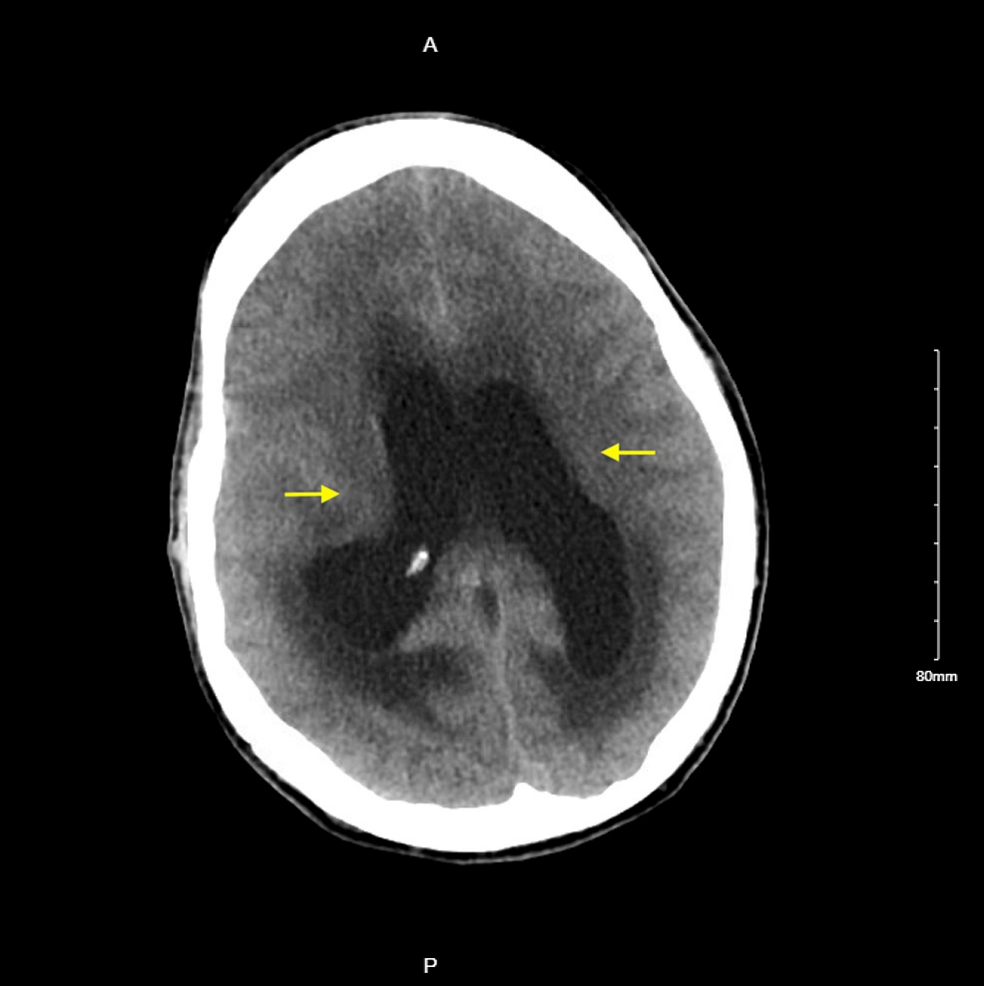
CT head on initial presentation: moderate dilatation of the lateral ventricles with marked subependymal edema (yellow arrows).
